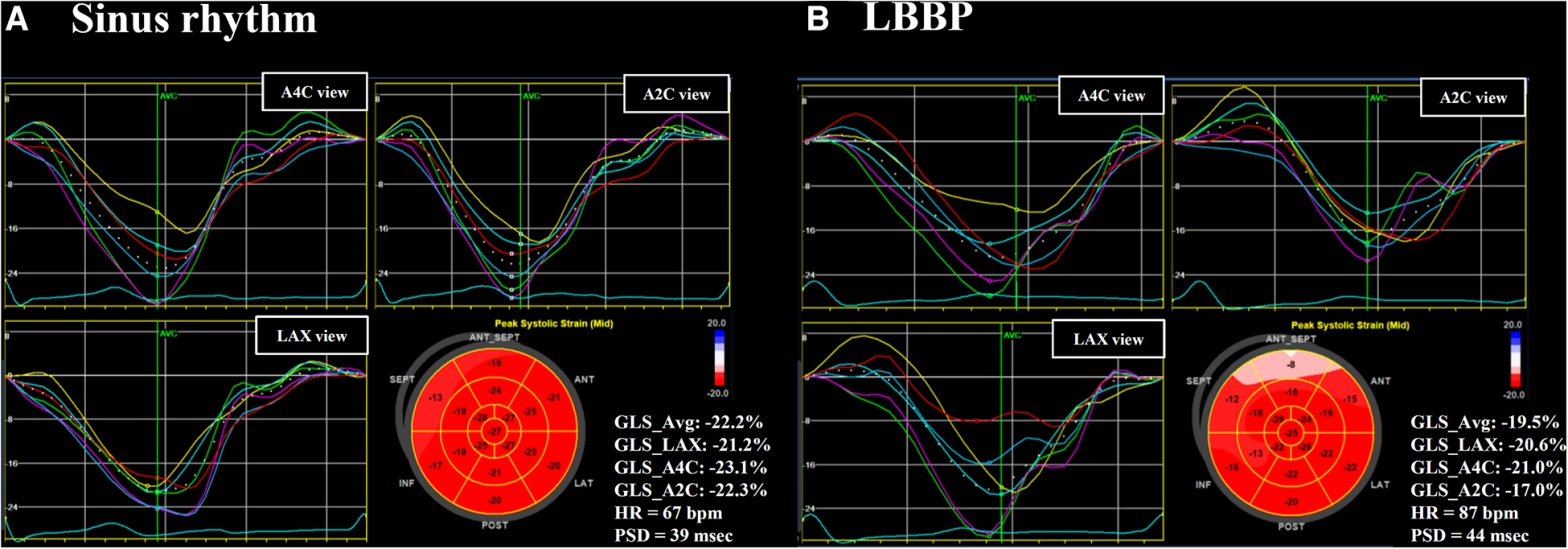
Left ventricular GLS and PSD. Left ventricular (LV) GLS and PSD assessed in a patient during sinus rhythm (A) and during LBBP (B). The value of GLS in apical three-chamber (LAX) view, apical four-chamber (A4C) view, and apical two-chamber (A2C) view were calculated individually. A bull's-eye plot was created of peak LV GLS for each myocardial segment. GLS, global longitudinal strain; HR, heart rate; LBBP, left bundle branch pacing; PSD, peak strain dispersion.
